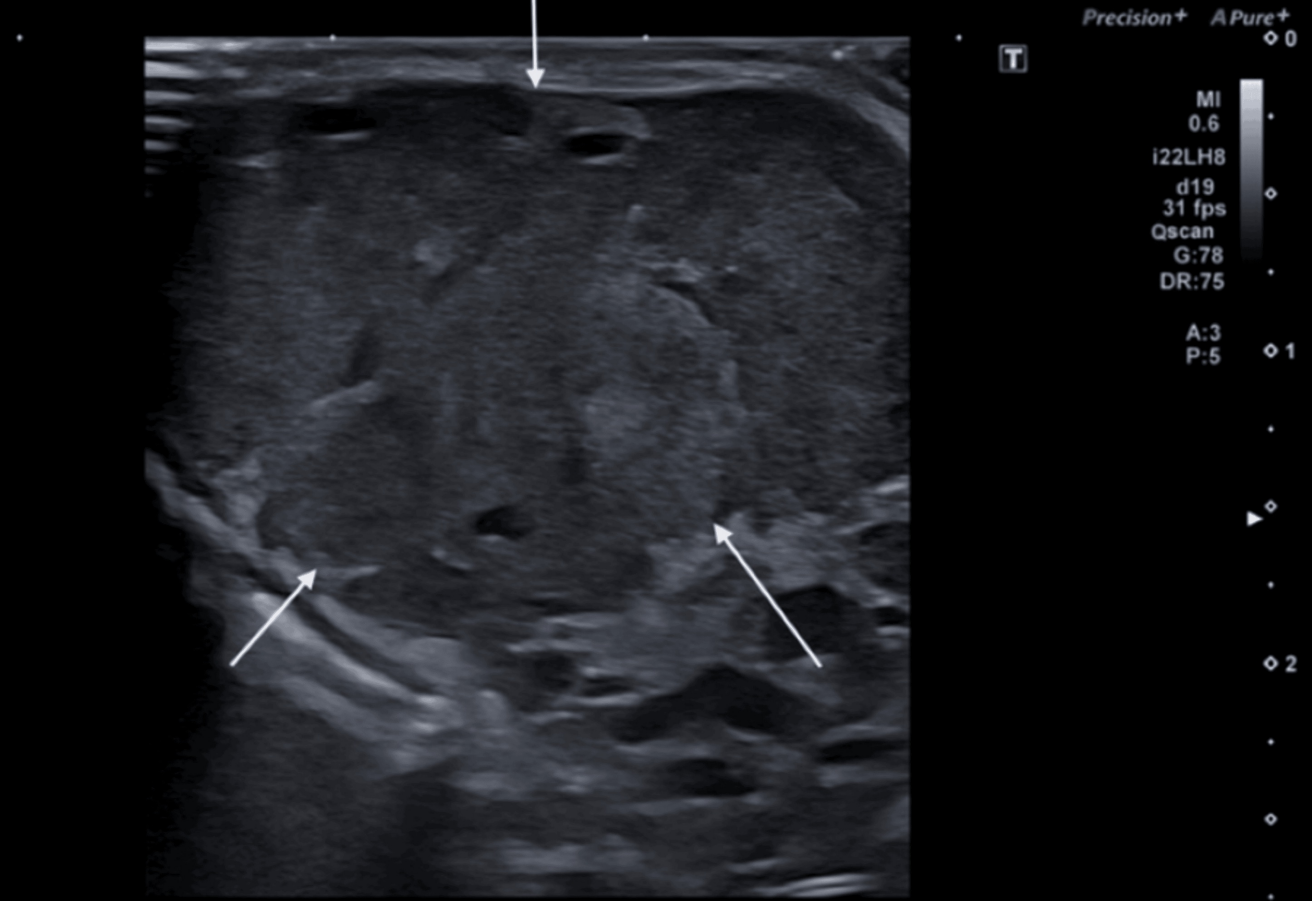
Ultrasound shows a sharply defined hypoechoic mass (white arrows) in the submandibular region.
